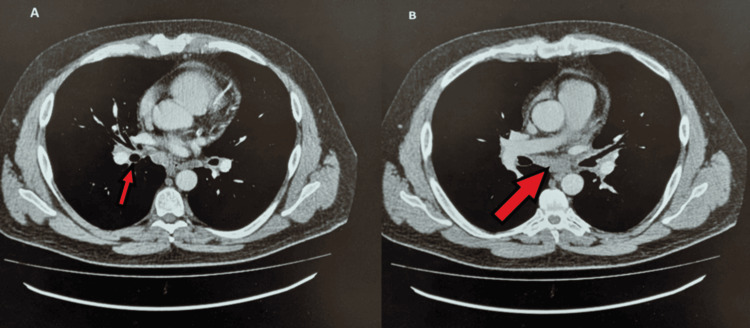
CT of the chest showing mediastinal (A) and hilar lymph nodes (B)
